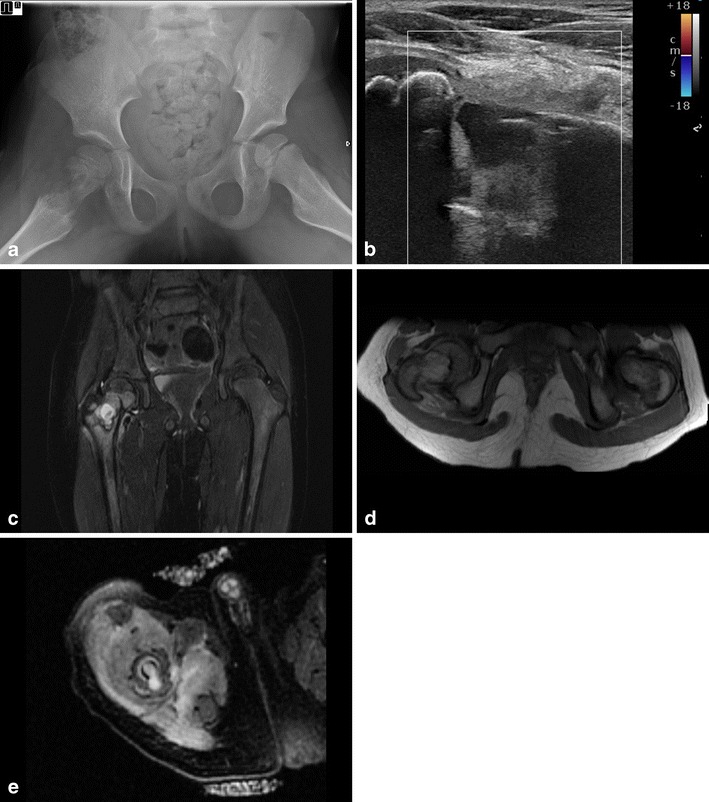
a A 3-month-old boy with a fever and reluctance to use his right limb. Physical examination shows a swollen, red hip. Conventional imaging shows extensive destruction of the proximal right femur and swollen soft tissue. b Ultrasound of the right hip shows cortical destruction, the formation of a subperiostal fluid collection/abscess and infiltration of soft tissue. c–d Coronal T1 STIR and axial PD images show cortical destruction and fluid collection/abscess formation in bone marrow. No contrast-enhanced imaging was performed in this case. e Axial T2 FS image confirms the fluid/abscess collection with extension outside the bone
